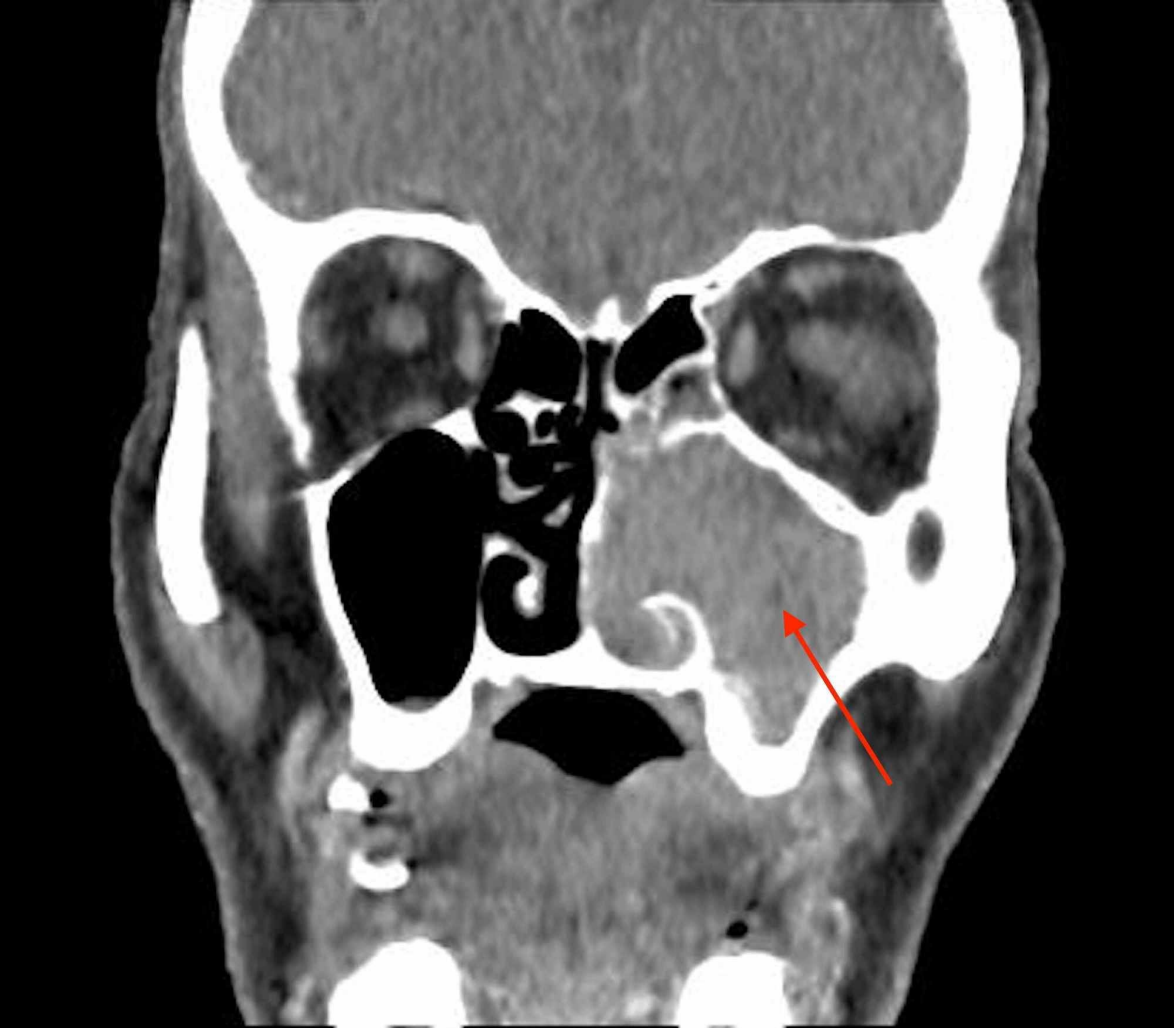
Coronal maxillofacial non-contrast computed tomography scan showing complete opacification of the left maxillary sinus & nasal cavity.
